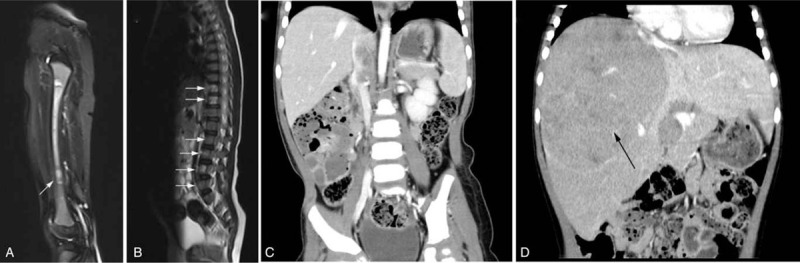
(A) Extensive hyperintensity and isointensity in the left femur by weighted MRI; (B) hyperintense in T10-11 thoracic, L2-L4 lumbar and S1 sacral vertebrae (white arrow); (C) no obvious mass was shown at diagnosis by contrast-enhanced axial CT; (D) a large heterogeneously enhancing soft-tissue mass (black arrow) in liver at relapse by contrast-enhanced axial CT.
